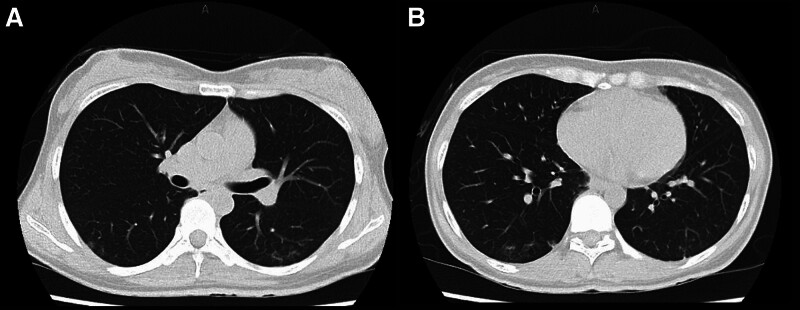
Computed tomography findings of the chest showing ground-glass opacity dorsally in both lower lung lobes. (A) At the level of segment 6 (S6). (B) At the lung bases.
